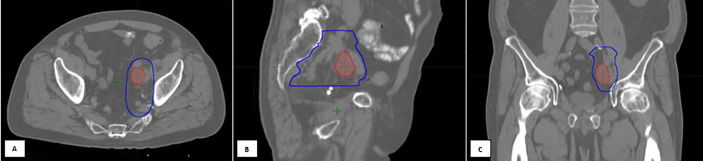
An 80-year-old gentleman with oligo-recurrent prostate cancer with left external iliac adenopathy identified on PET imaging [(A) Axial, (B) Sagittal, (C) Coronal views]. The decision was to proceed with SBRT assisted Intermittent ADT. An involved field SBRT approach was utilized. The left external iliac, left obturator, and left internal iliac nodal regions were treated with 27.5 Gy in 5 fractions (high risk CTV), with a 30 Gy simultaneous integrated boost to the gross disease. Treatment planning computed tomography images showing the isodose lines for the GTV prescription of 30 Gy (red) and the High-risk CTV prescription of 27.5 Gy (dark blue line). Following SBRT, induction ADT was initiated.
